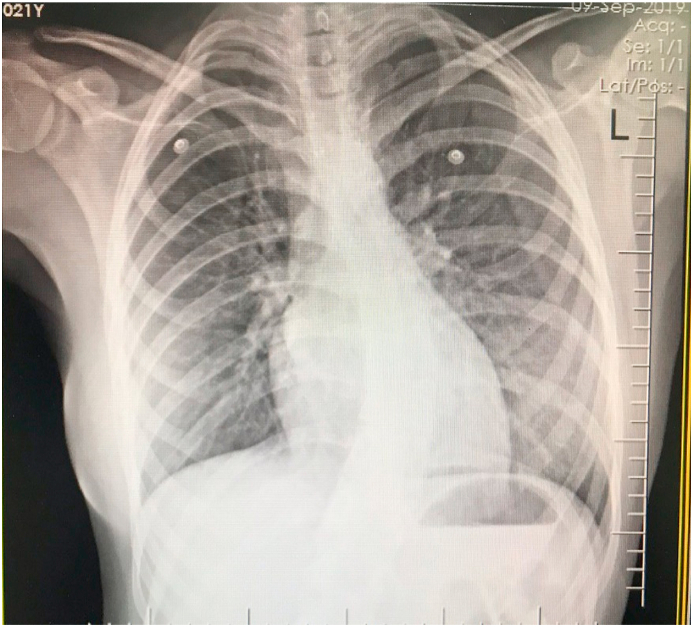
Pulmonary echocardiogram with scoliosis in patient.
